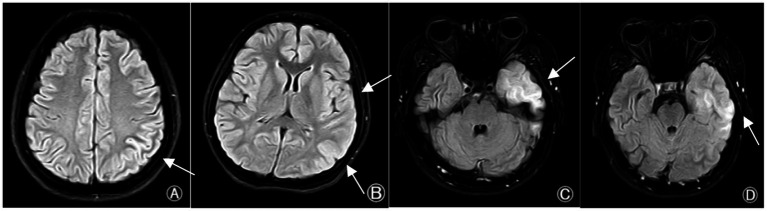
Cranial MRI findings in the acute phase of MOGAD with seizures as the initial symptom in adults. (A) In Case 1, there is slight swelling of the left parietal lobe cortex. (B) In Case 6, there are abnormal FLAIR hyperintensities in the left temporal lobe and occipital lobe. (C,D) In Case 7, there are FLAIR hyperintensities in the local cortex of the left temporal lobe.
